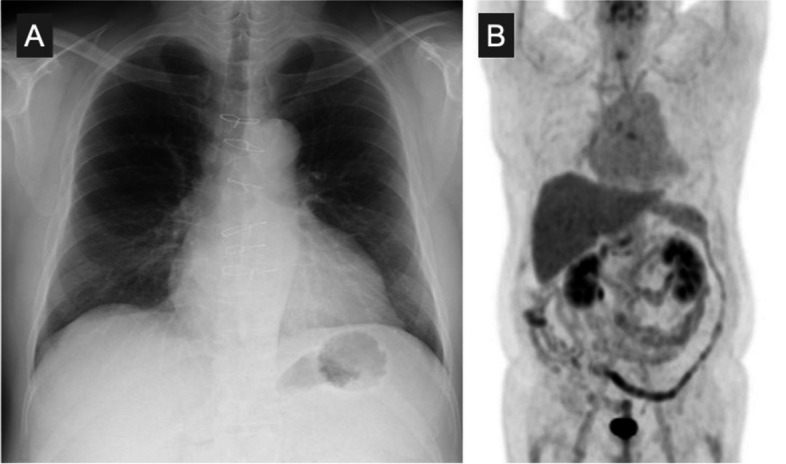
Postoperative image at 10 years after resection. A Chest X-ray shows that the cardiac silhouette shrinks down into the normal range. B Positron emission tomography reveals no abnormal accumulation
